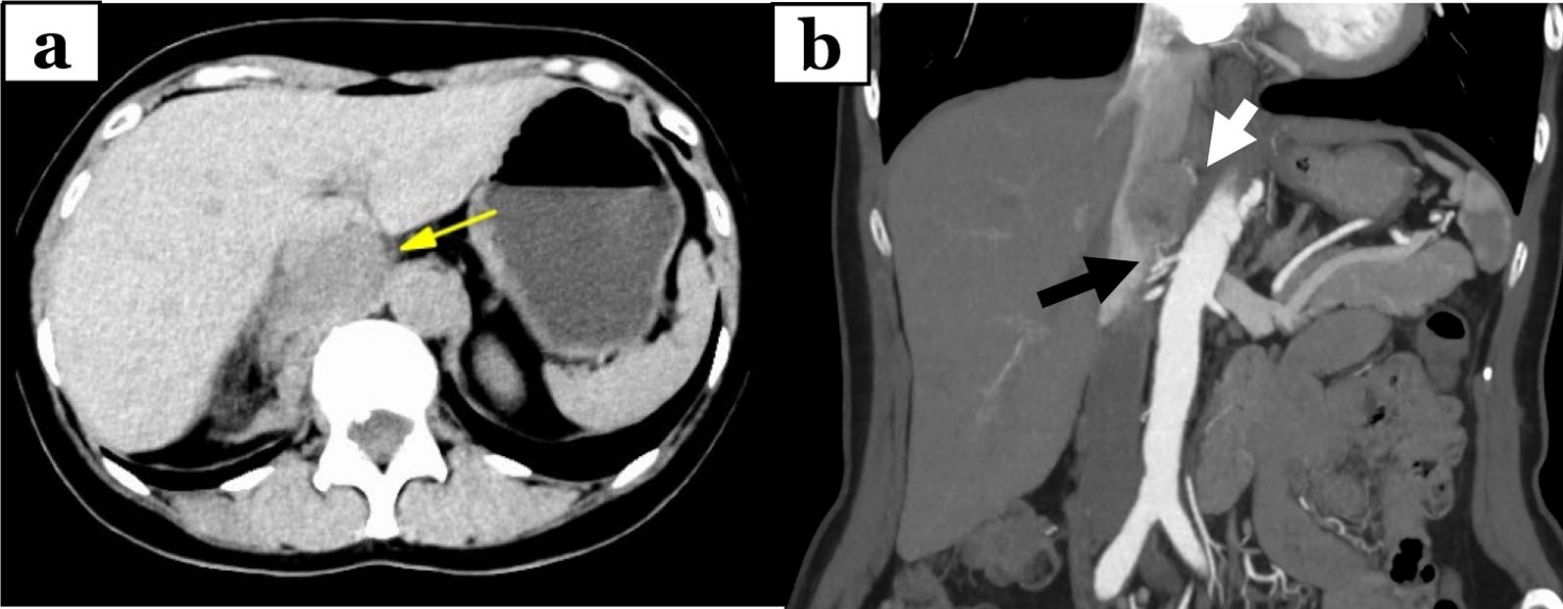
Computed tomography findings at the time of emergent admission. a Computed tomography (CT) showed an enlarged mass and surrounding retroperitoneal hemorrhage. b CT showed that the tumor was dominated by two arteries
